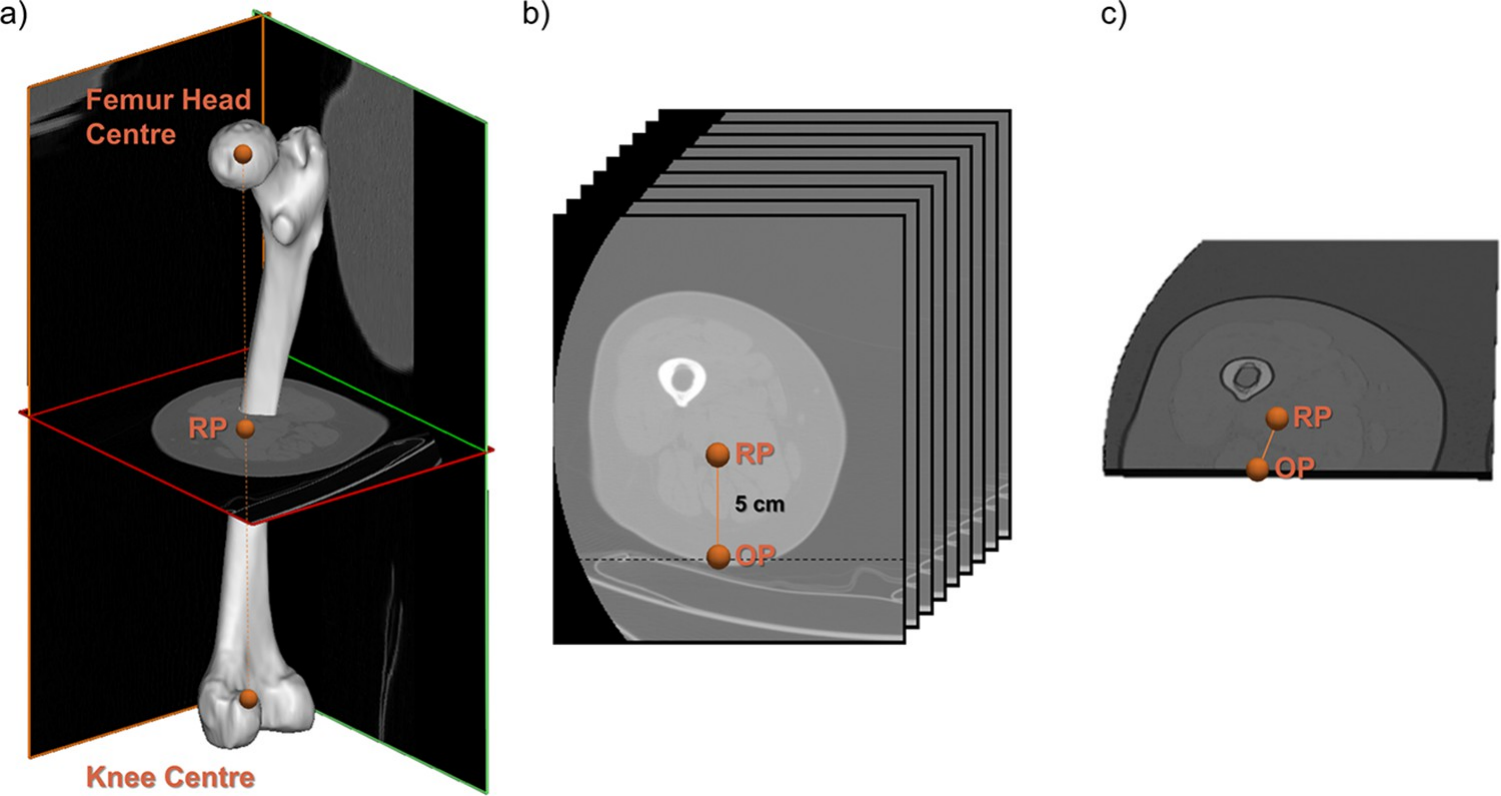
Definition of VOIcut from CT scan slices. (a) RP definition based on the Femur Head Centre and Knee Centre. (b) VOI definition based on RP and OP by removing the portion extending 5cm posteriorly from the RP. (c) VOIcut selection. The visualization of the CT scan was obtained from the data collected during the study, which is available at [42].
